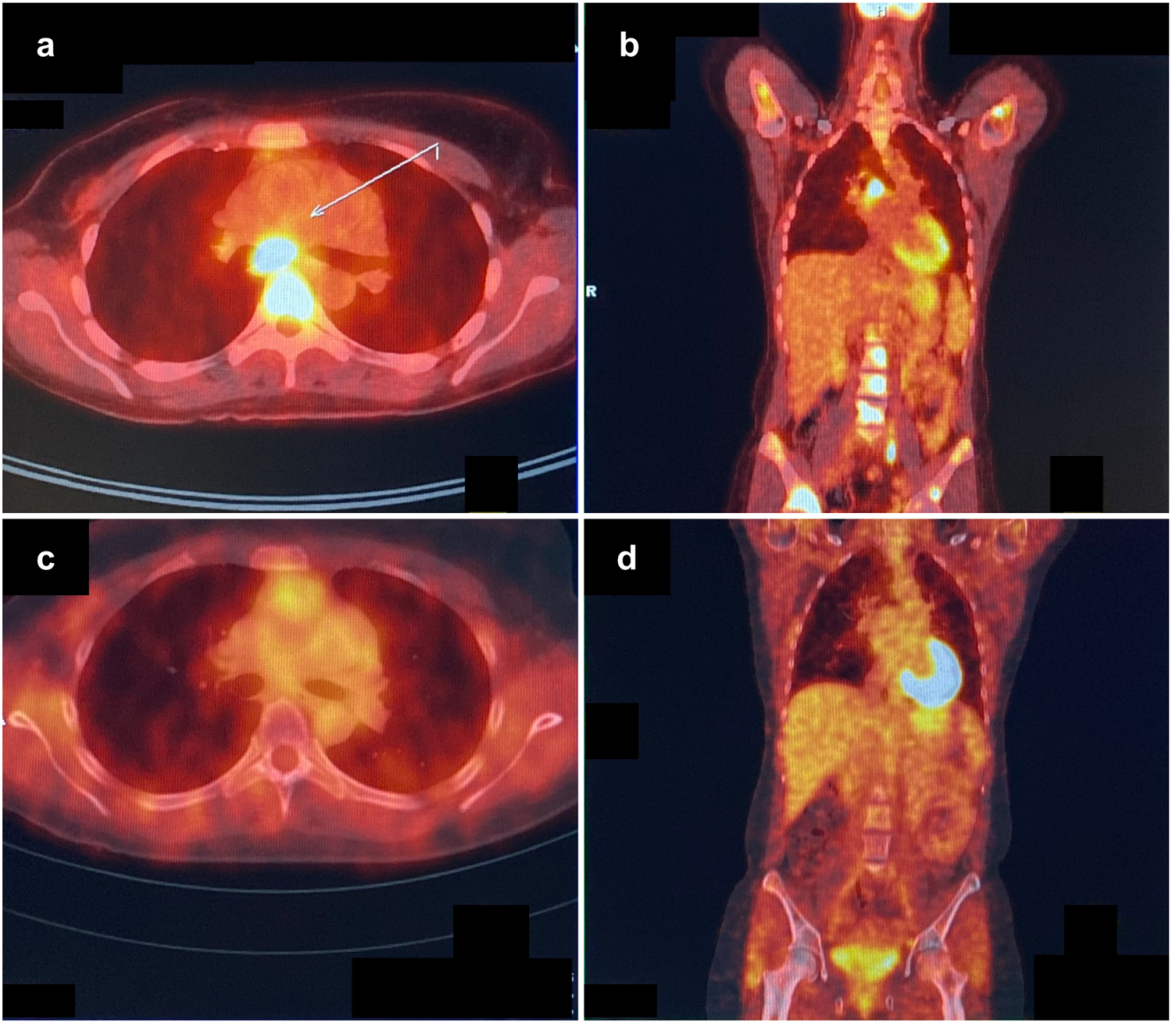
Radiologic response to olaparib. Axial (a) and coronal (b) PET/CT images acquired prior to initiation of olaparib show hypermetabolic mediastinal lymph nodes and multifocal bony attachment in the spine and pelvis. After 7 months of olaparib therapy, axial (c) and coronal (d) PET/CT images indicate significant remission of lymph node and bone metastases.
